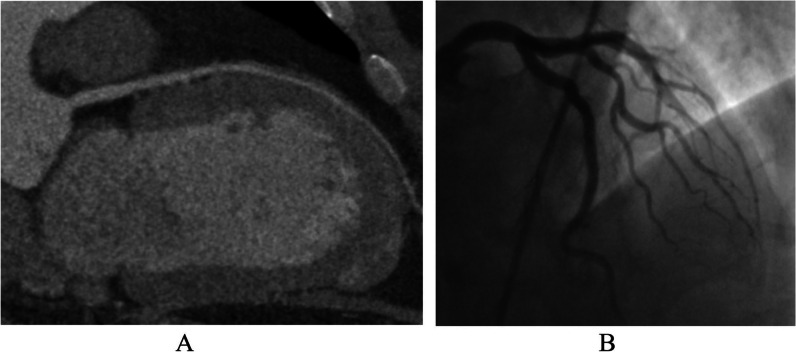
Patient imaging examples. Example of negative coronary CT (A) and negative ICA (B). A Multiplanar reconstruction of the left main and left anterior descending of a patient randomized to coronary CT angiography without pathological findings, CAD was excluded. B Patient example of normal invasive coronary angiography showing strong vessels of the left coronary artery and its branches of the left anterior descending and left circumflex artery, CAD was excluded
